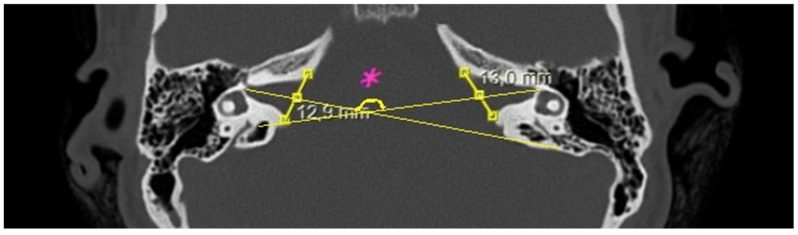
Axial temporal bone CT showing IAM orientation. The long axes of the IAMs (lines) form the orientation angle (°) (indicated with an asterisk), which may influence posterior fossa surgical exposure.
